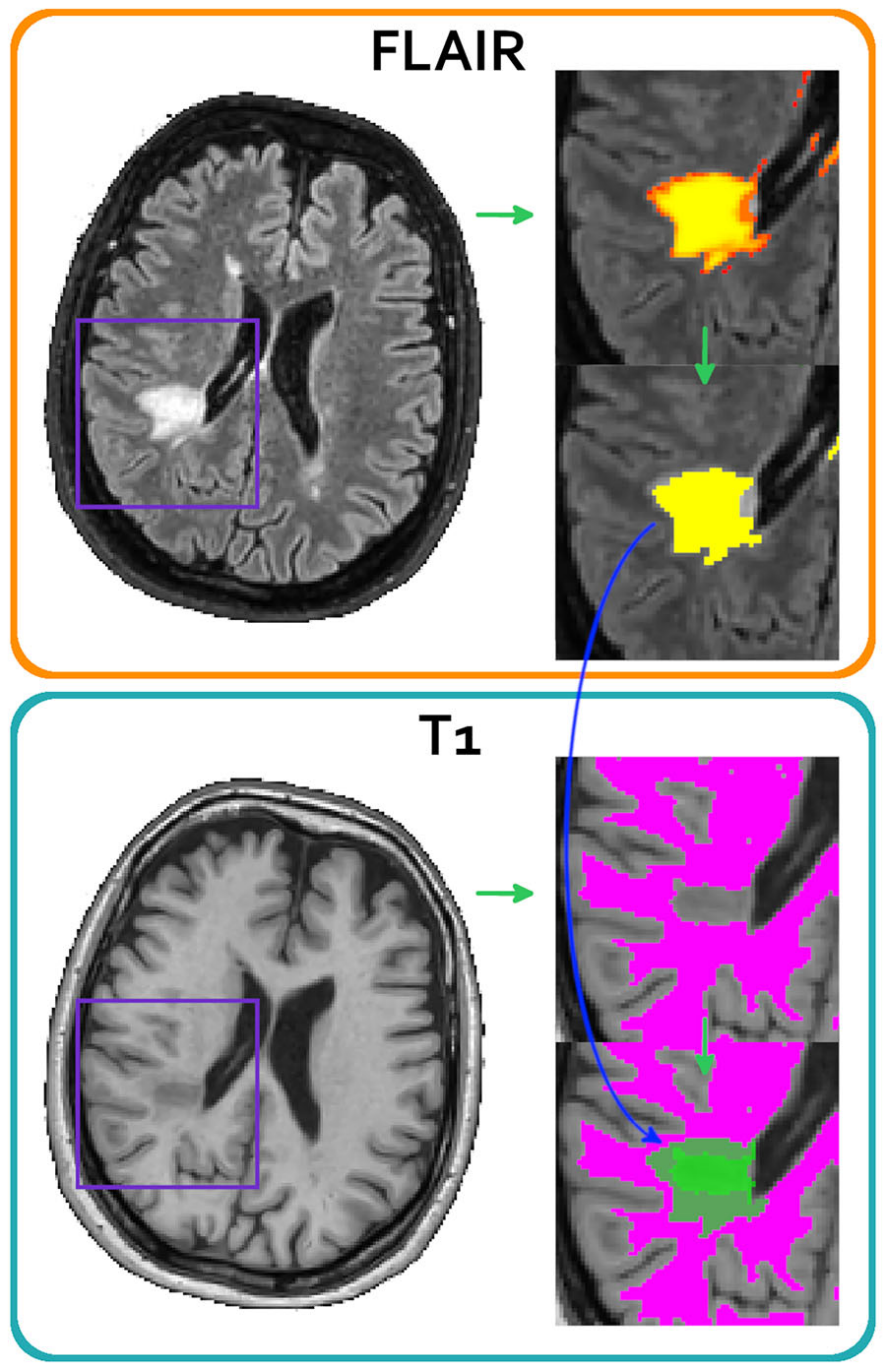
Lesion segmentation process on FLAIR and T1 images. Lesions identified with Lesion Segmentation Tool (LST) on FLAIR images (orange box) are represented with a probability map (top of the zoomed view); according to LST recommended usage, only the area characterized by a lesion probability >0.5 has been kept (yellow area, bottom of zoomed view). The cyan box shows white matter (WM) segmentation, where the lesion is not included in the non‐lesional WM mask (top of the zoomed view); at the bottom of the zoomed view, it is shown the lesion mask overlaid onto the WM mask: Hypo‐ and iso‐intense areas are visible as two shades of green.
